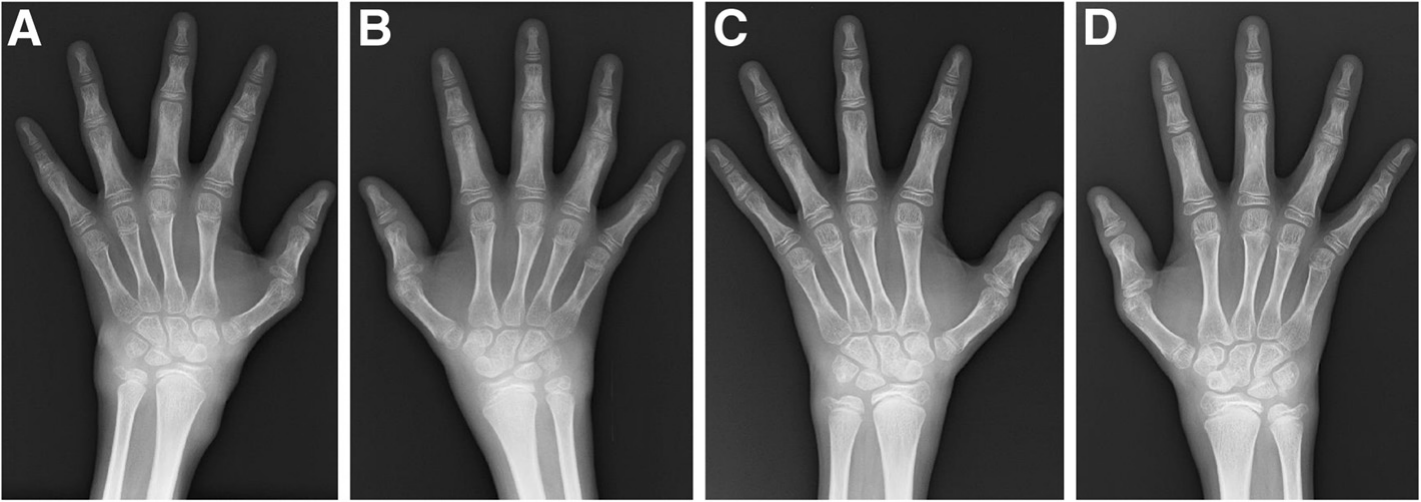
Radiographs of the hands in patient 5. Shown are the radiographs of the left hand (A, C) and the right hand (B, D) in patient 5 before treatment (A, B) and after treatment (C, D). Soft tissue swelling in the wrist and fingers improved after treatment with methotrexate and infliximab.
